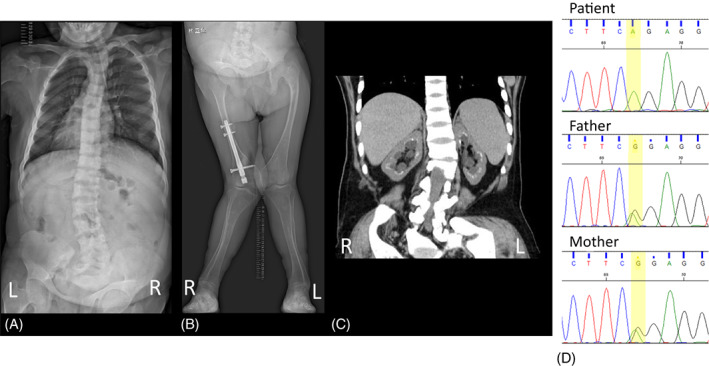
Radiographs (A), (B) and abdominal CT (C) at 43 years of age. (A) A spinal radiograph of the back indicating scoliosis. (B) Lower extremities exhibited genu varum. (C) Abdominal CT indicates bilateral kidney calcification in the corticomedullary region. Direct sequencing indicated the presence of a homozygous *SLC7A7* variant (NM_001126105.3:c.1417C > T,p.R473*) in the patient and a heterozygous variant in her parents.
